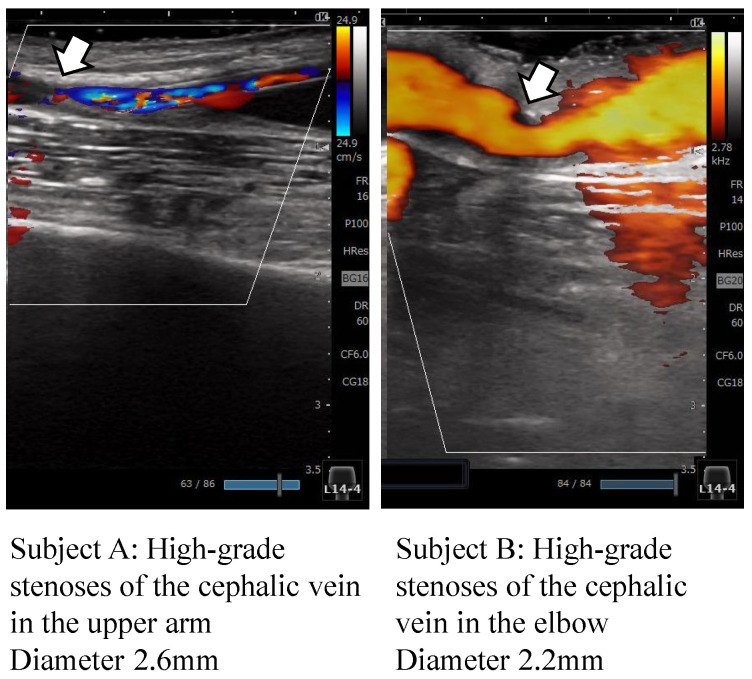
Ultrasound echocardiographic stenosis.
